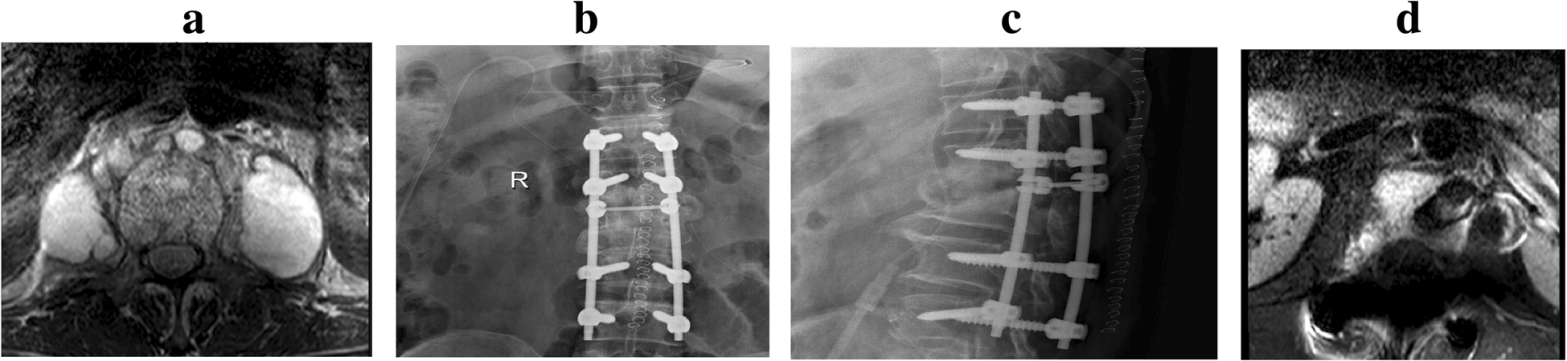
A 39-year-old male patient with tuberculosis of the L1/2 lumbar vertebrae and PA underwent posterior pedicle screw fixation and anterior debridement, with bone graft fusion. a. Preoperative MRI showed lumbar tuberculosis, with abscess formation. b, c. The X-ray image 3 days after surgery showed good internal fixation and bone grafting. d. Postoperative MRI at the 3-month follow-up showed complete debridement and no obvious abscess
